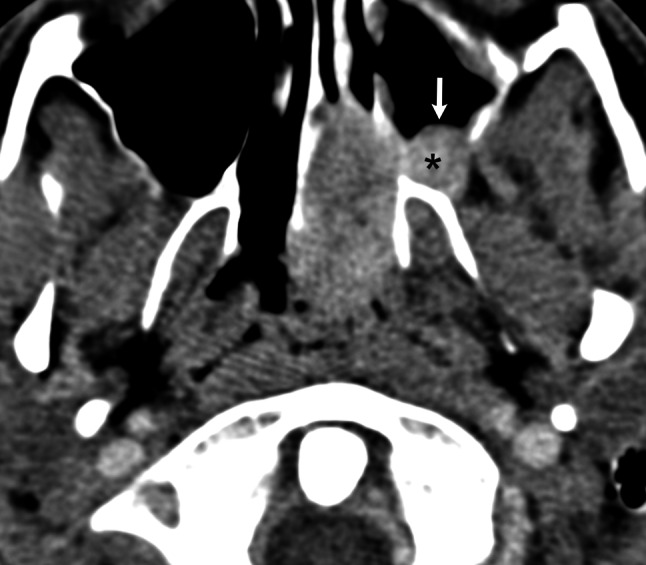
Contrast-enhanced axial CT scan indicates forward displacement of the posterior maxillary wall (*arrow*) and tumor invasion of the pterygopalatine fossa (*asterisk*)
